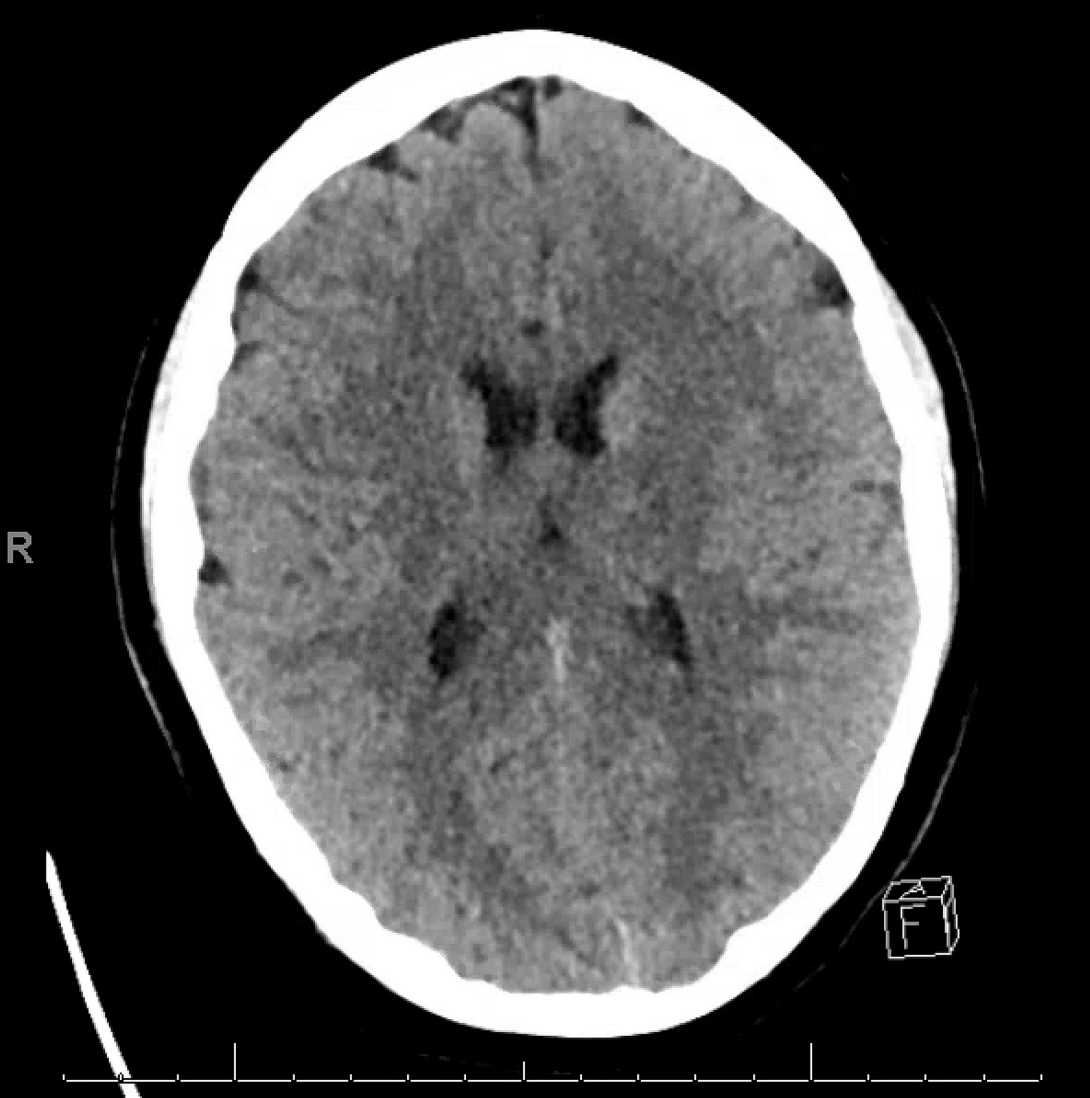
CT of brain at admission with bilateral symmetrical foci of hypodensity within the hypothalamic regions, basal ganglia and thalami bilaterally
